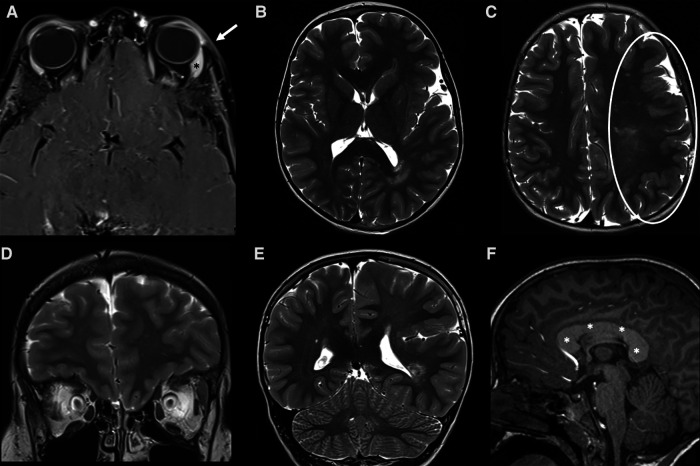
MRI of a 7-year-old Male child with left-sided facial hypertrophy with multiple reddish macules on the face and left orbit, with a clinical suspicion of macrocephaly-capillary malformation (M-CM). (A) A slight thickening associated with subtle enhancement of the skin and subcutaneous tissue (white arrow) of the left orbit is noted on fat-sat post-contrast T1 images, corresponding to one of the clinically visible macules. A slight hypertrophy of the left lacrimal gland is also noted (asterisk). (B–E) Axial (B,C) and coronal (D,E) images of the brain revealed a marked asymmetry of the cerebral hemispheres, with left being larger than right consistent with left hemimegalencephaly. The cortex of the enlarged hemisphere is also diffusely polymicrogyric (white circle in C). (F) on the post contrast sagittal T1 an abnormal thickness of the entire corpus callosum is also evident (white asterisks). A mosaic mutation of PIK3CA gene was discovered, confirming the clinical suspicion of M-CM.
